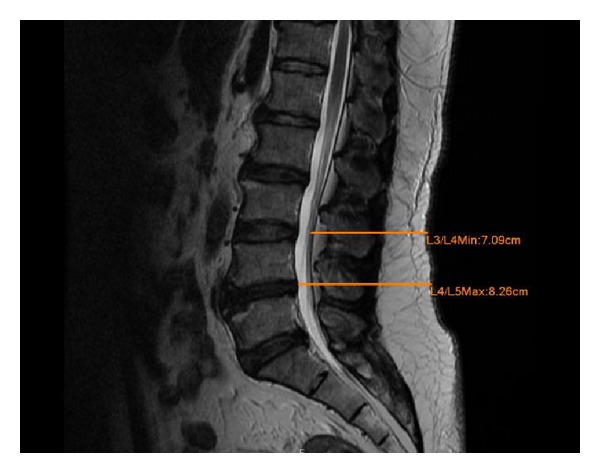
MRI of L-spine. Upper line is the posterior margin of the spinal canal to the skin (Min). Bottom line is the anterior margin of the spinal canal to the skin (Max).
